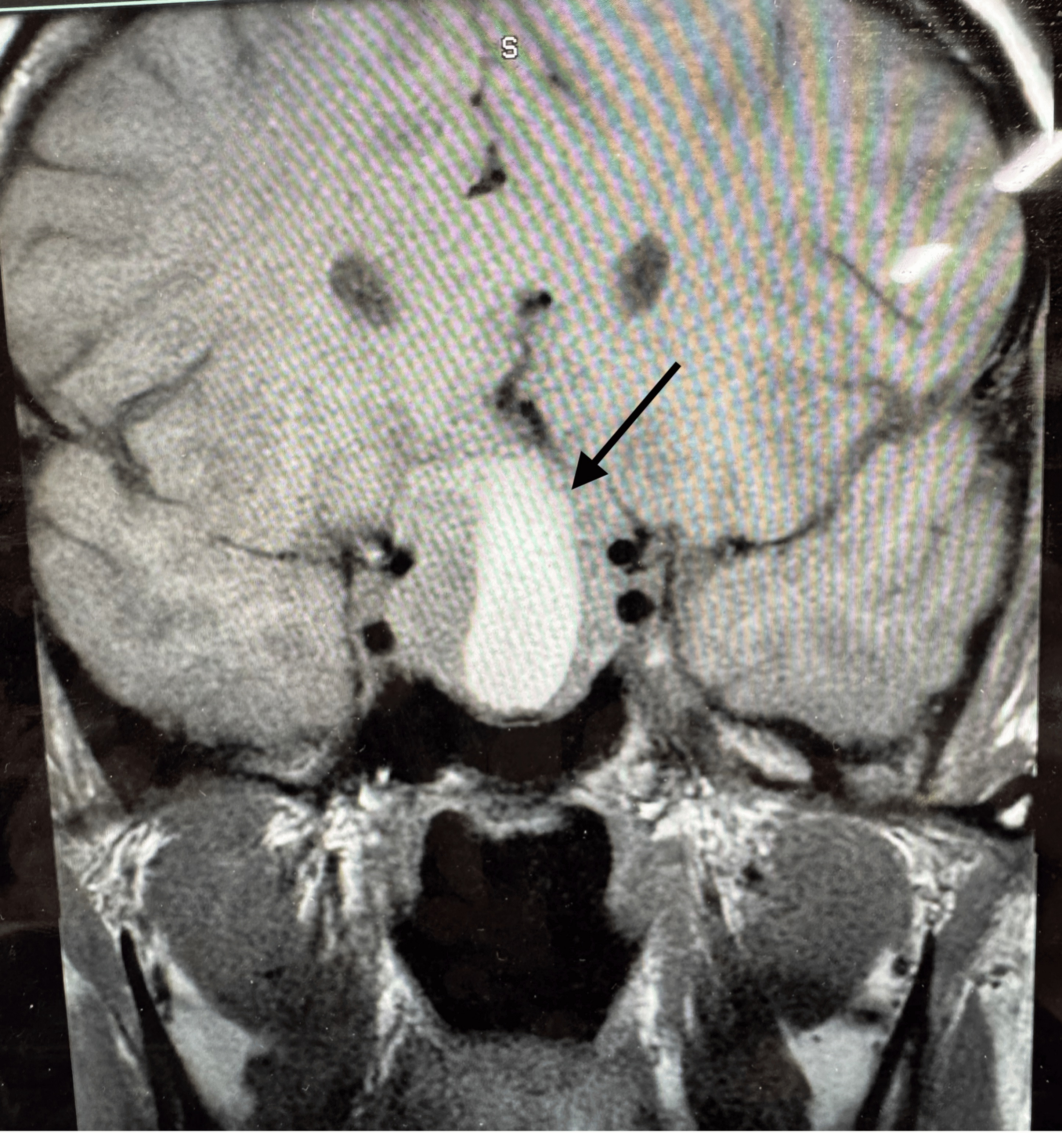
MRI of the pituitary five days after treatment showing a pituitary mass measuring 30 × 25 × 20 mm. MRI: magnetic resonance imaging
